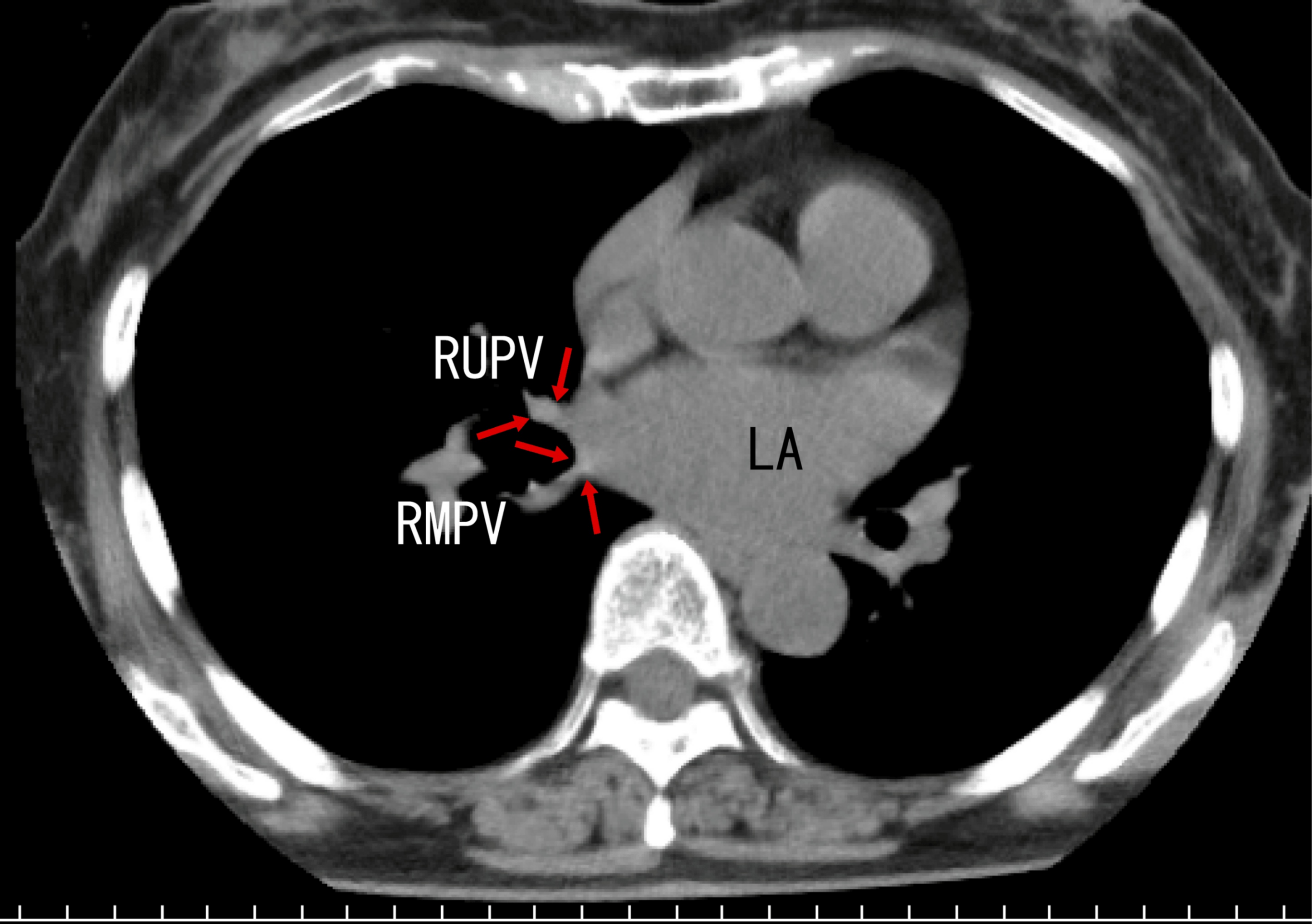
After three months of dabigatran treatment, axial plain CT images After three months of dabigatran treatment, axial images revealed calcification around the exit of the RUPV and RMPV as white areas (arrows). Compared with Fig. 4, the white areas decreased to some extent, especially around the exit of the RUPV. LA: left atrium, RMPV: right middle pulmonary vein, RUPV: right upper pulmonary vein
